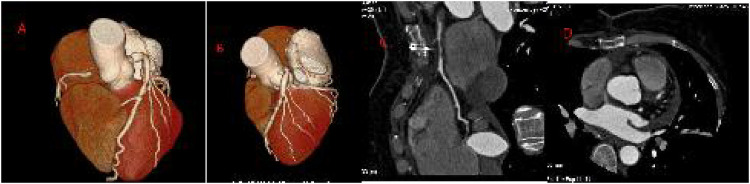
Coronary CTA at 2 months after surgery. VR-reconstructed three-dimensional images show the proximal occlusion of the right coronary artery and the collateral circulation network of LCA; CPR sagittal view shows the proximal occlusion of RCA; Axial four-chamber view shows the proximal occlusion of the RCA.
